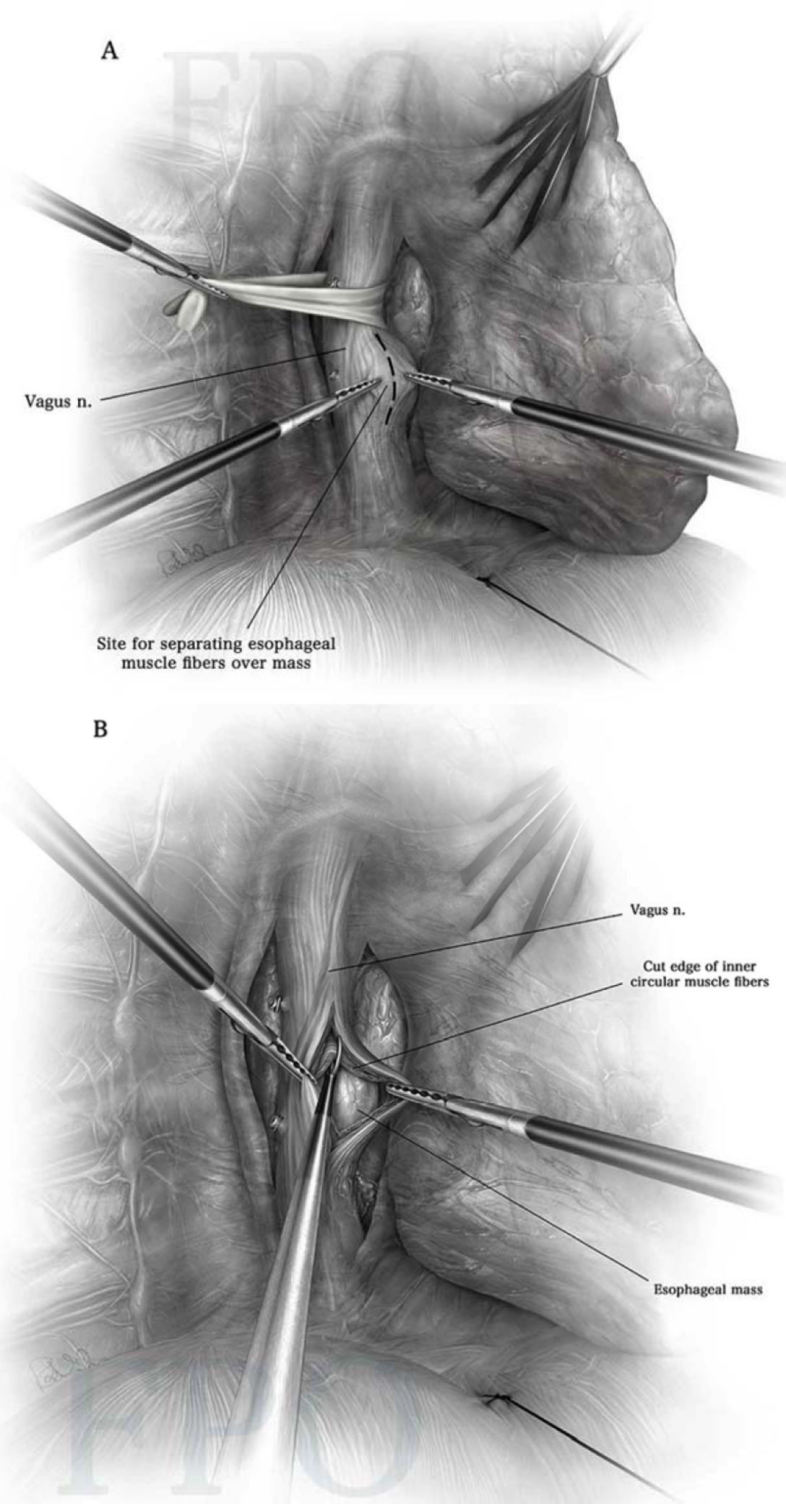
A&B. The lesion is then located by direct vision and palpation. If the lesion is difficult to find, intraoperative upper endoscopy may be performed to aid with localization. Passage of a bougie may also help to exaggerate the location of the lesion by displacing it radially. Distraction with either the endoscope or a bougie is highly recommended, as large lesions can cause transluminal protuberance, and the wrong side of the esophagus may be entered. A myotomy is then performed directly over the lesion. The outer longitudinal muscle is separated (not divided) with a blunt pulling technique, hook cautery, or ultrasonic shears. The inner circular muscle fibers are then divided sharply with hook cautery or ultrasonic shears to expose the lesion. Unlike a Heller myotomy where the muscle may be quite thickened, the muscle fibers overlying the lesion are often attenuated and separate easily. The myotomy is carried proximally and distally only as far as needed to identify the borders of the mass. The vagus nerves should be identified and preserved. The vagus nerve can be mobilized and swept away to allow for extension of the myotomy beneath the path of the nerve if necessary.
